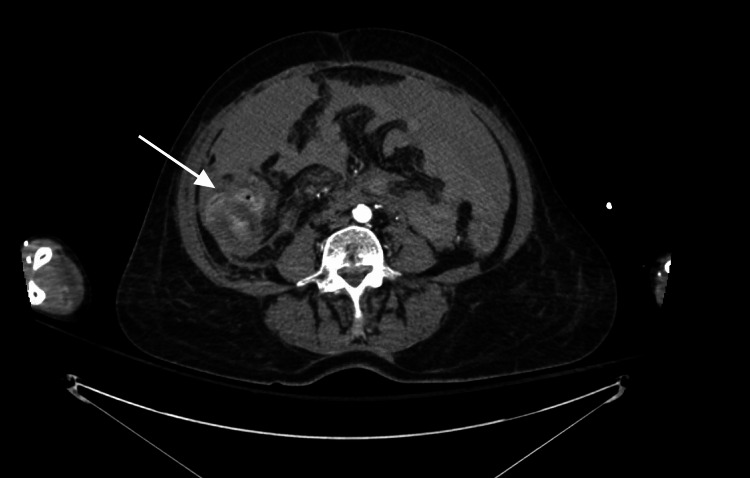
A repeat CT scan demonstrated ongoing active contrast extravasation into the right peritoneal cavity and the lumen of the ascending colon, along with progressive hemoperitoneum (arrow).
